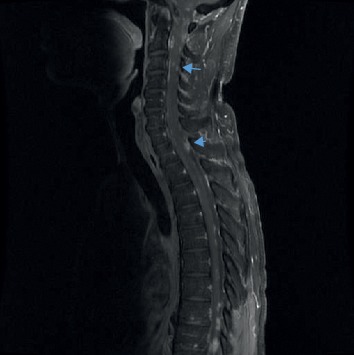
MRI spinal cord, sagittal, T2-weighted showing hypointense nodules localizing to the level of the inferior endplate of T2 and C7 (blue arrows).
